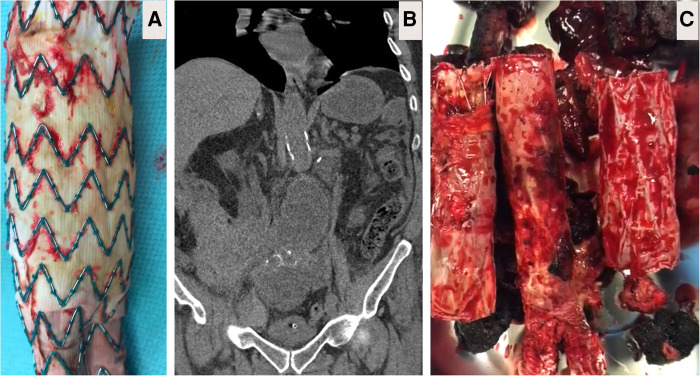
Explantation. (A) Body of partially explanted Cook (Bloomington, IN, USA) polyester-based endograft depicting micro-fabric pores due to chronic fatigue failure close to the allies of the stent. (B) Computed tomography angiography (CTA) image showing partially explanted Cook graft with the suprarenal spare springs and hooks left in-situ. (C) Here, patient underwent primary endovascular aneurysm repair (EVAR) with Endologix AFX (Endologix, Irvine, CA). However, this polytetrafluoroethylene (PTFE) based endograft failed due to the fracture of the endoskeleton, which acted as a hinge against the PTFE. Attempts to salvage the EVAR resulted in implantation of two proximal cuffs over six years follow-up. The patient presented with rapidly expanding 8 cm abdominal aortic aneurysm and abdominal pain, necessitating immediate total graft explantation and aorto-bi-iliac reconstruction by 16 × 8 mm silver Dacron graft (Maquet, Rastatt, Germany).
